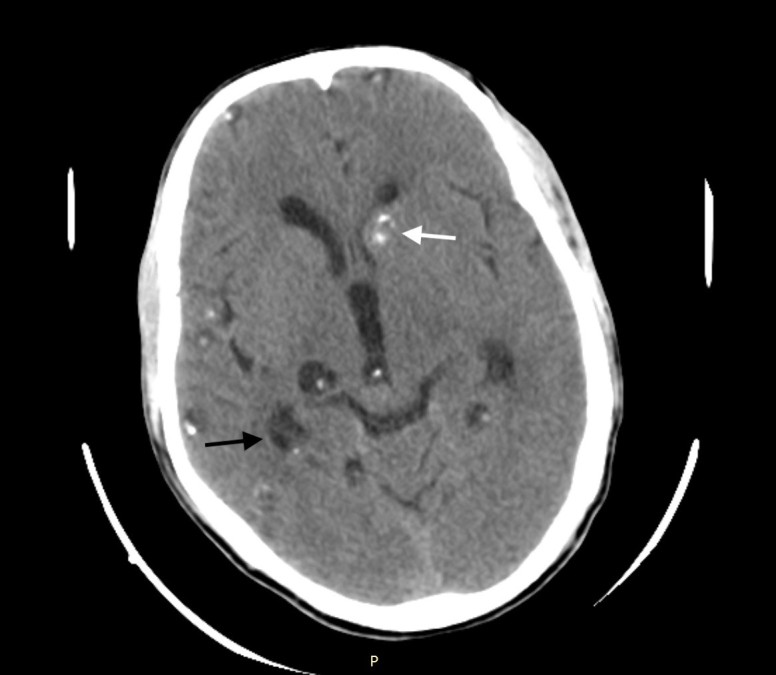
Computed tomography of the brain showing multiple cystic lesions in different stages: vesicular (black arrow) and nodular calcific stage with lesion in left cerebral hemisphere near basal ganglion showing calcification (white arrow).
